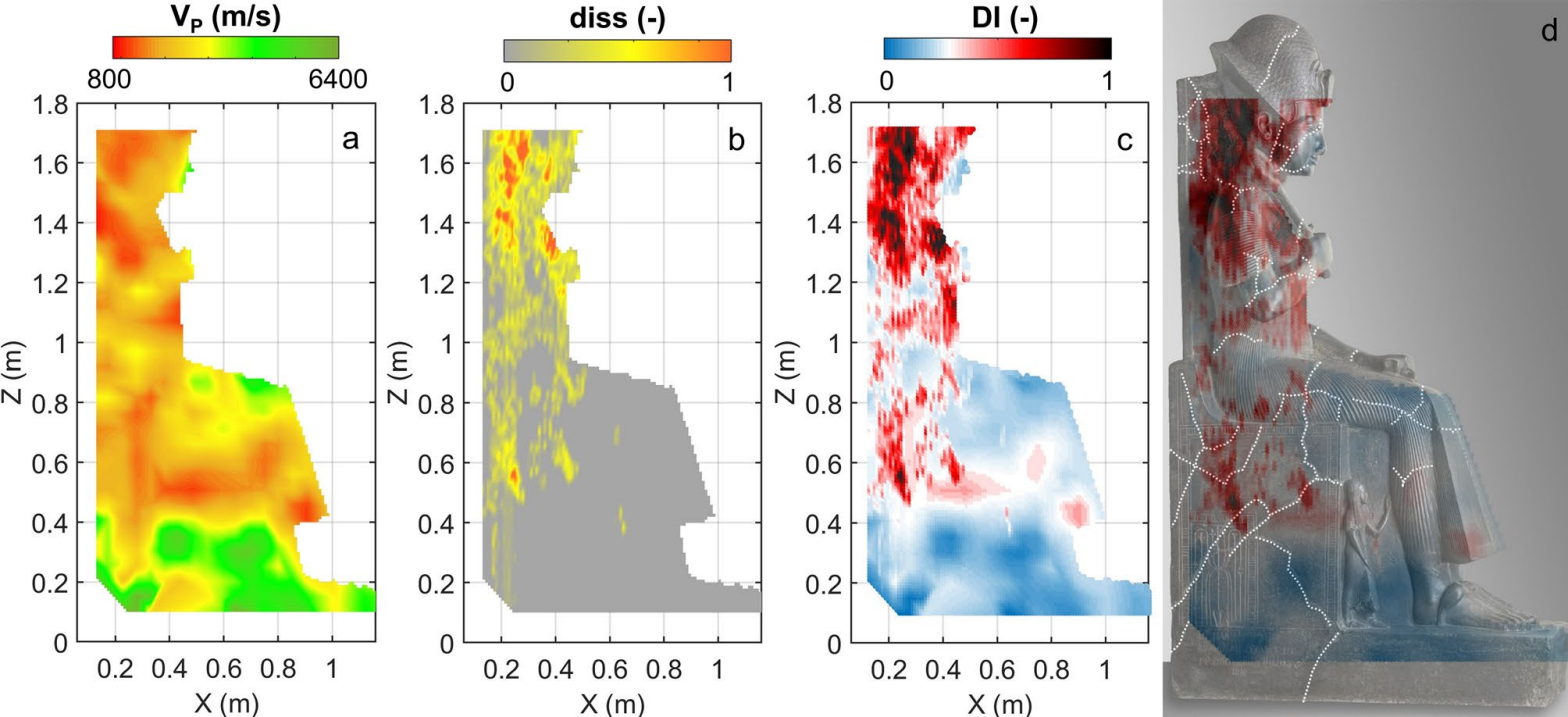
(a) Ultrasonic velocity, (b) local dissimilarity (L11 scan), (c–d) damage index on the axial vertical section of the statue of Ramses II. In (d) superficial fracture traces are highlighted on the image of the statue by white dashed lines. Figure created in Matlab (version R2021b, https://it.mathworks.com/products/matlab.html).
